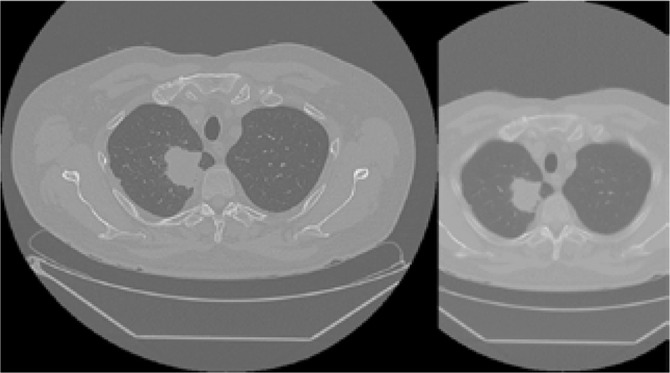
Thoracic CT scan showing a tumoral mass in the upper lobe of the right lung.
